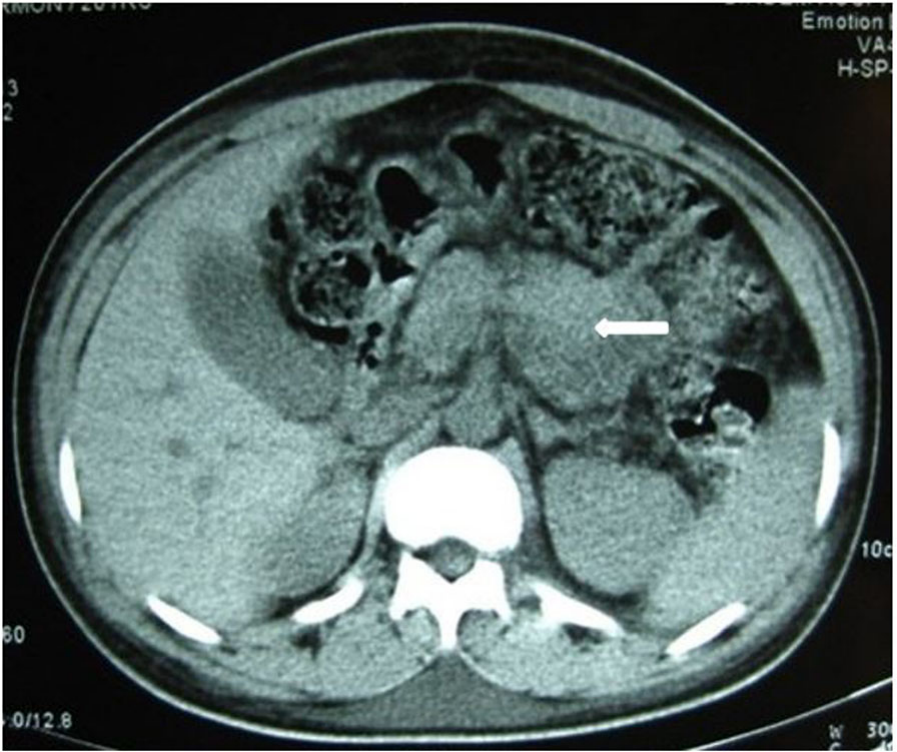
Non-contrast-enhanced computed tomography of the patient’s abdomen shows swollen pancreas (*white arrow*) with peripancreatic edema suggestive of acute pancreatitis
